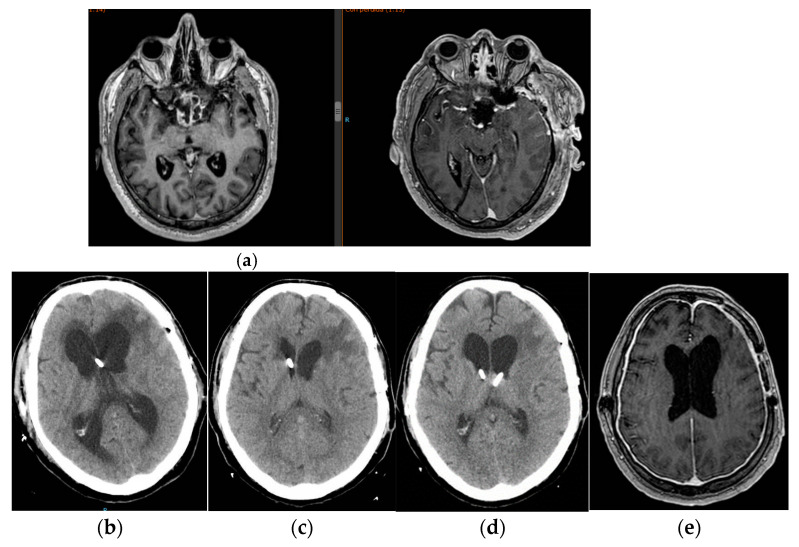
Image (a) pre-surgical and immediate post-surgical images of patient n 5 (b): development of hydrocephalus being treated with EVD. Image (c): normalization of ventricular size at day 25 after subzero drainage. Image (d): new ventricular dilatation despite definitive shunt treated by a new wide external catheter in left ventricle. Image (e): magnetic resonance imaging at discharge. Hyperdrainage signs are seen with ventricular size almost normalized.
